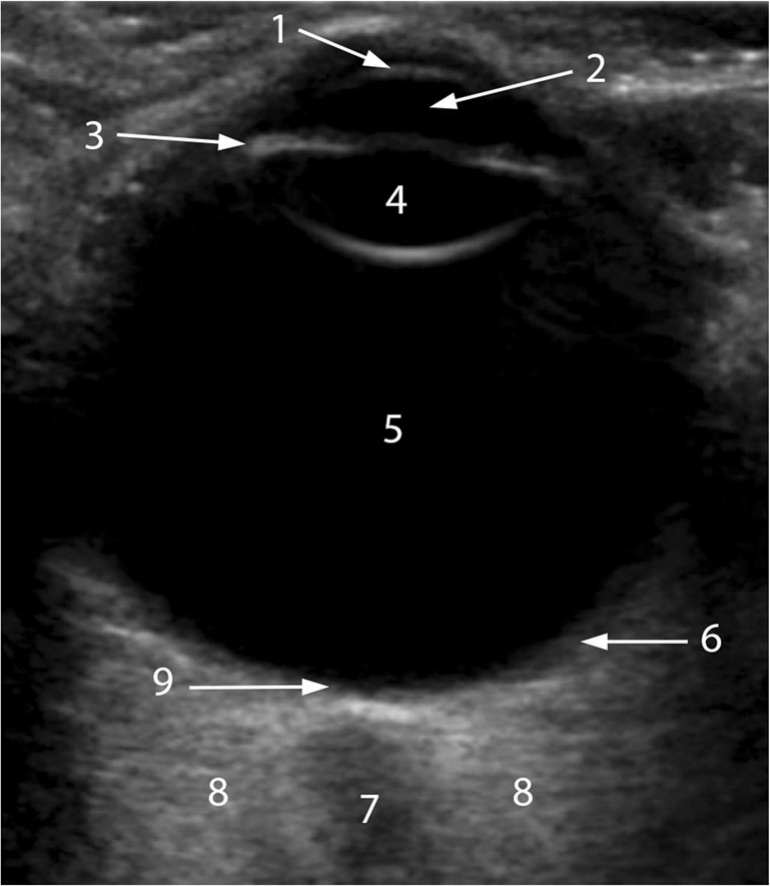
Sonographic appearance of the structures of the normal eye. The cornea (1) is visualized as the most superficial echogenic curved line; the anterior chamber (2) is anechoic. The iris (3) appears as a thin echogenic line. The lens (4) is defined by anterior and posterior boundary echoes, but the lens itself is echo-free. The vitreous chamber (5) is filled with a clear gel-like substance that is normally echo-free, although the formation of spots and linear echoes with aging is considered normal. The RCS complex (6) forms the wall of the posterior ocular segment; it is seen as an echogenic concave line extending from the iris plane to the optic nerve (ON; 7). The ON is seen as a hypoechoic band surrounded by echogenic retrobulbar fat (8). The circular area where the ON connects to the retina is the optic disc or papilla (9)
